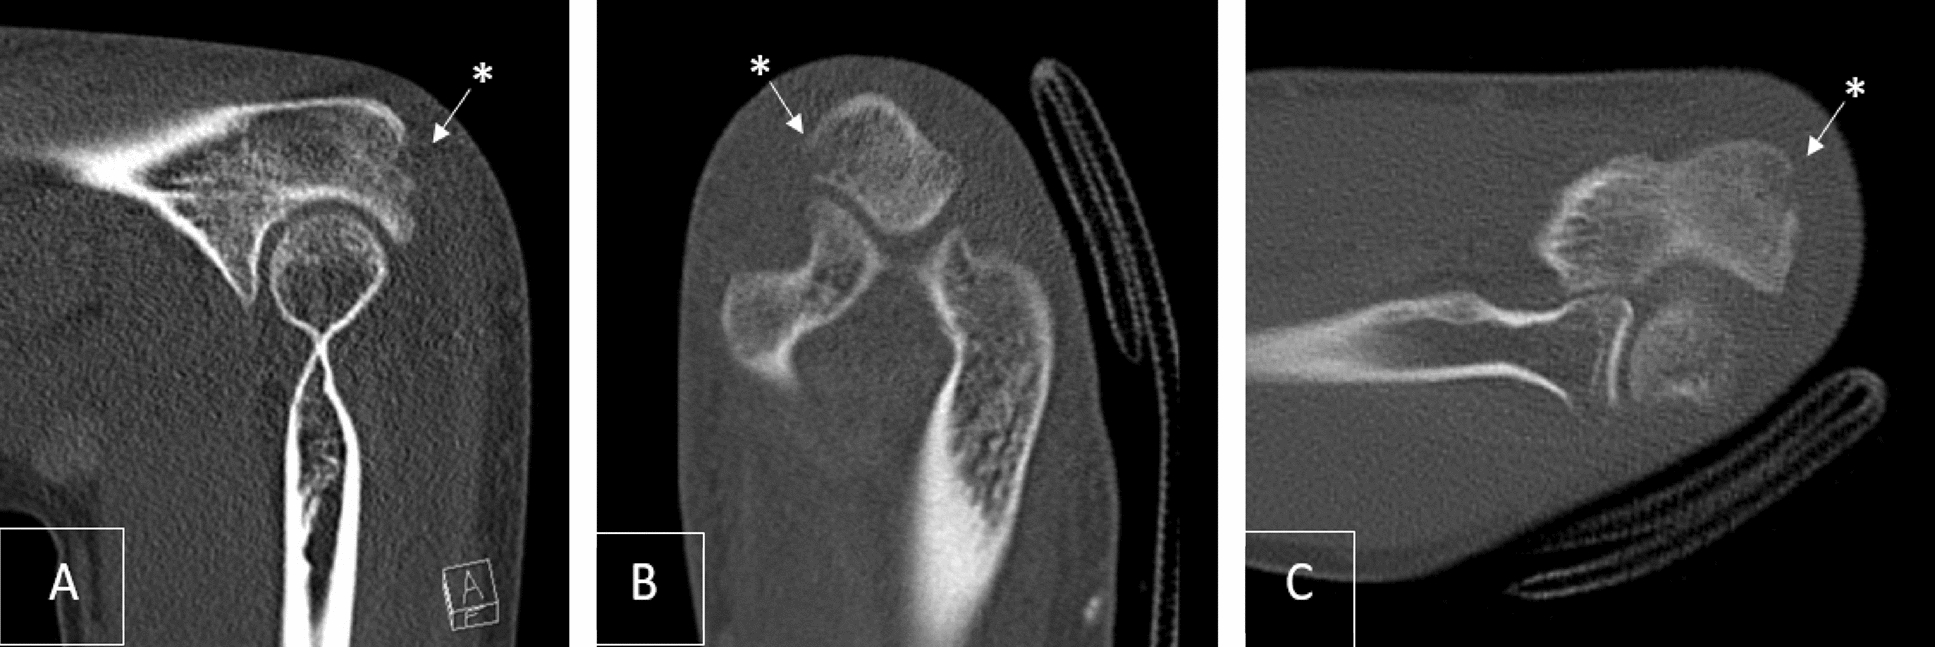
Computed tomography scan of the left olecranon; A sagittal; B axial; C coronal; *shows suspected erosion of the olecranon
